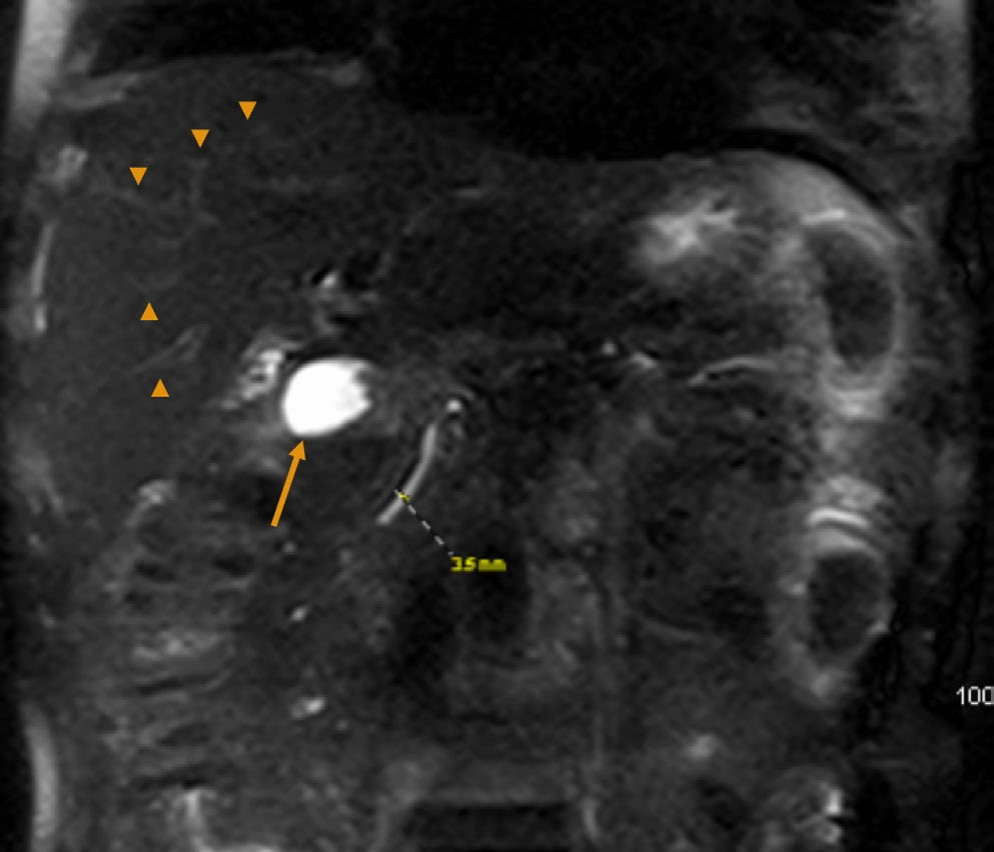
Noncontrast MRI/MRCP after admission showed normal liver parenchyma without metastatic lesions. No apparent intrahepatic ductal strictures (arrow‐heads). The gallbladder (long arrow) has multiple nonobstructing gall stones with no pericholecystic fluid, wall thickening, or ductal dilation (dashed line). No apparent hepatic hilar lymphadenopathy (not shown)
